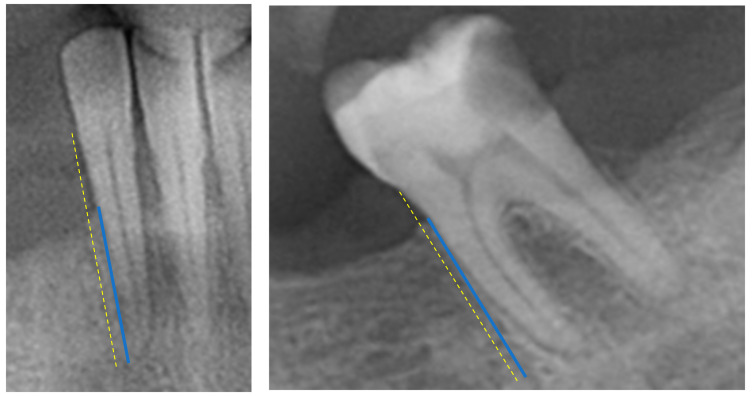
Radiograph-based Periodontal Bone Loss (R-PBL) was measured from the marginal alveolar bone to the tooth apex (blue line) and from the cementoenamel junction to the tooth apex (yellow dotted line).
